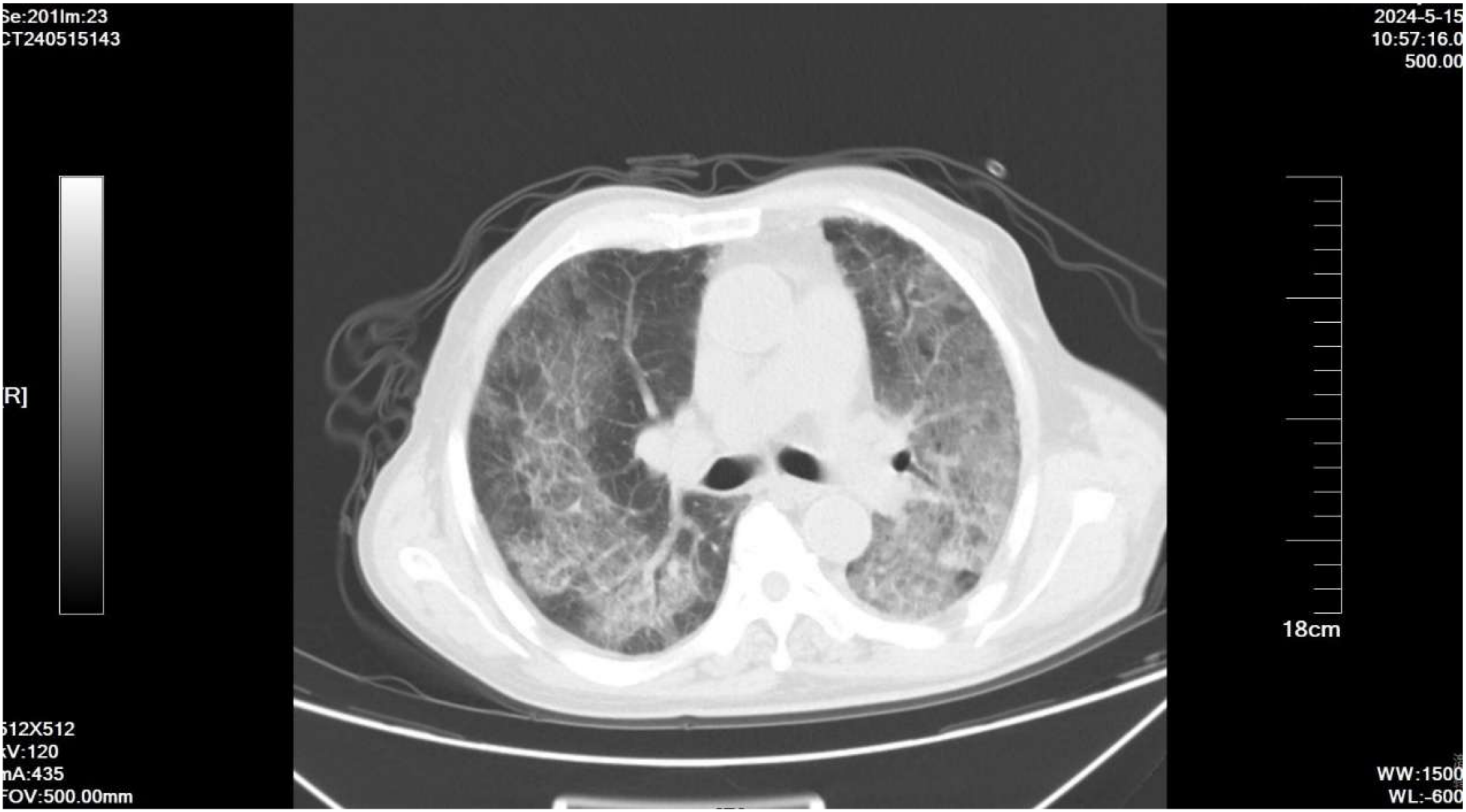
HRCT of the chest obtained 15 days after the first dose of nab-PTX revealed diffuse exudative changes in the bilateral lung parenchyma, accompanied by extensive ground-glass opacities and consolidation, involving more than 90% of the bilateral lung fields.
